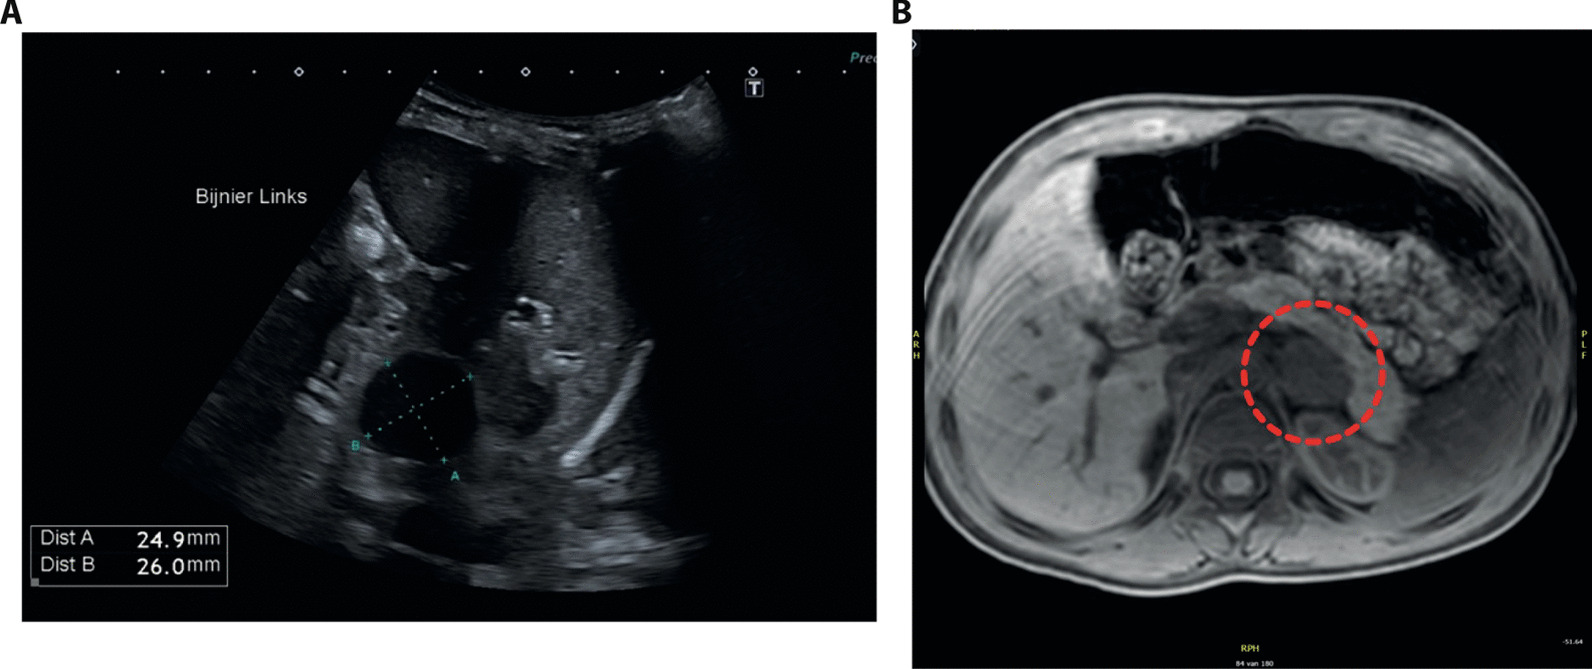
Imaging studies. A Abdominal ultrasound showed a hypoechoic lesion in the left adrenal. B MRI confirmed the presence of a well-defined 26 × 23 × 31 mm nodule (dotted red circle) with limited but homogeneous contrast accumulation, and no evidence of invasion into adjacent organs or vessels
